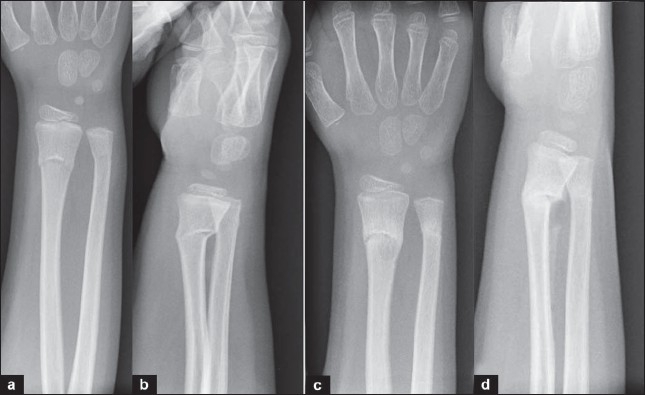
Ateroposterior(a) and lateral(b) X-rays of the forearm with wrist shows Greenstick fracture of the distal radius, with dorsal angulation. Anteroposterior (c) and lateral (d) xray at 3 weeks follow up shows faintly defined cystic area within the subperiosteal callus, just proximal to the fracture site.
